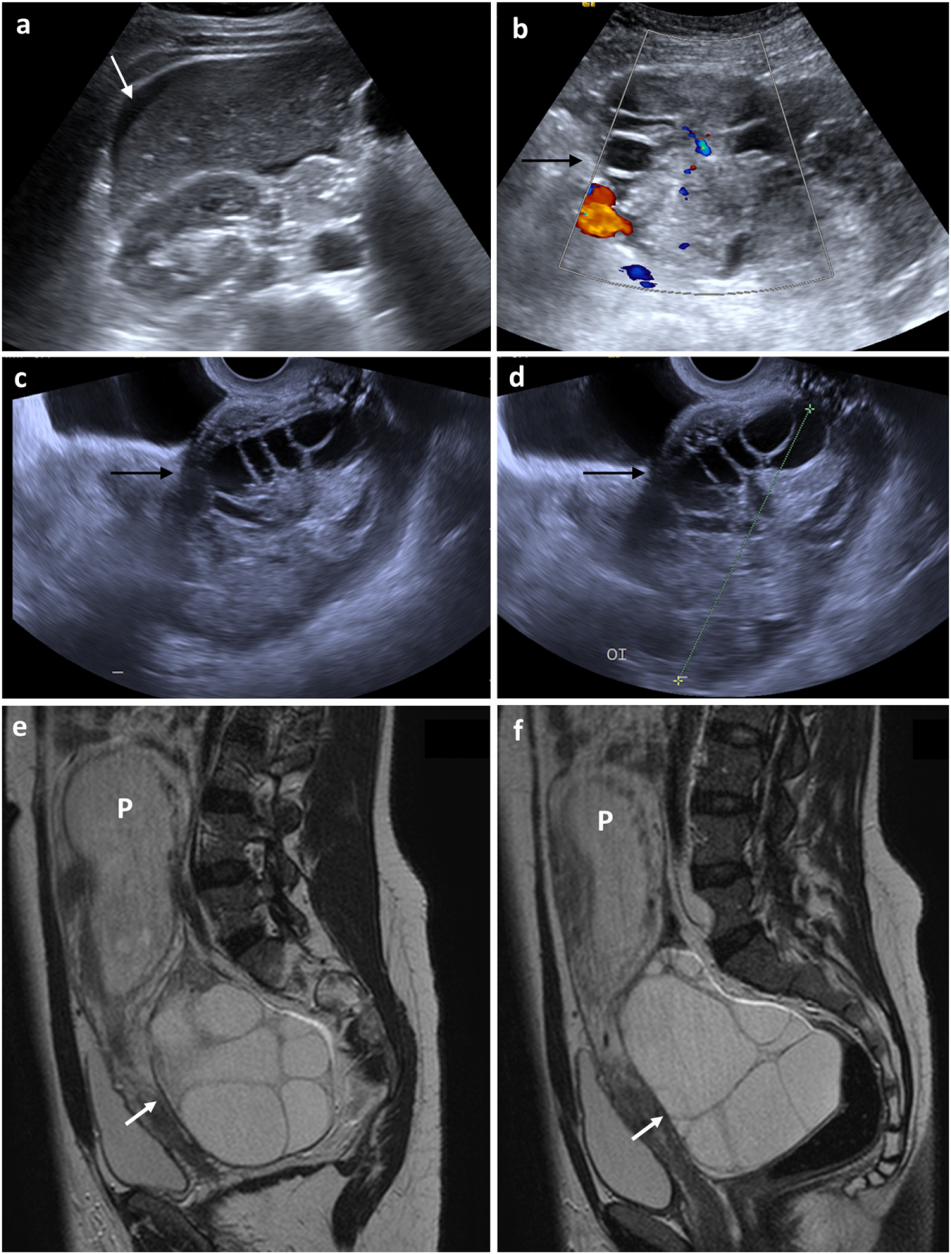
Ovarian hyperstimulation syndrome in two different patients. a–d Women in her 30’s undergoing in vitro fertilisation presented with abdominal distention, nausea and vomiting. TAUS (a, b) and TVUS (c, d) demonstrate ascites (arrow in a), enlarged ovaries (black arrows) with preserved central flow (b) and multiple follicles of varying sizes (b–d) consistent with OHSS. e, f Different patient in her early 30’s and 15 weeks pregnant presented with abdominal pain. Sagittal T2WI at the level of the right and left adnexal regions show markedly enlarged bilateral ovaries (arrows) containing multiple large, thin-walled cysts consisted with OHSS. P (placenta). MRI can provide better characterisation of the ovarian process in the differential diagnosis of ovarian tumours
